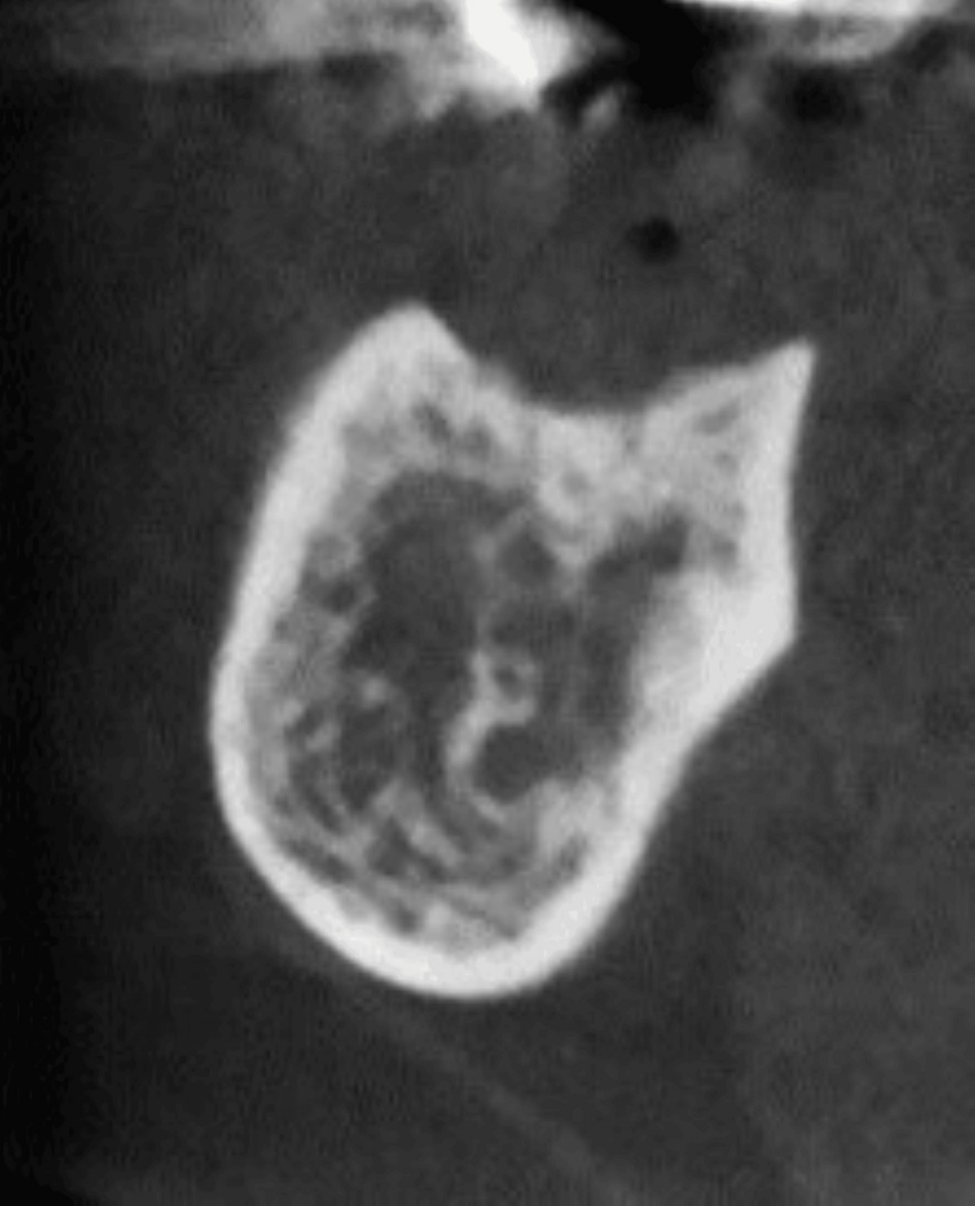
Case 8 There were no abnormal findings on CT images after the second surgery. However, four marginal resections were required before healing was achieved.
